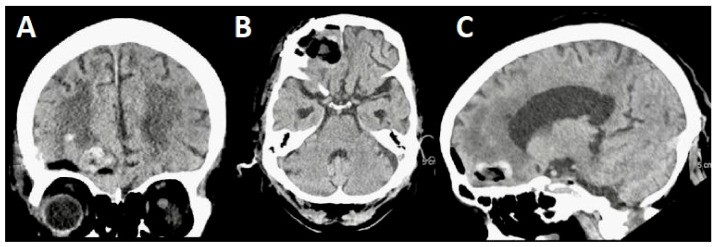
Case 2’s postoperative day 1 MRI showing a small amount of blood in the resection cavity with minimal compression of the frontal lobe. (A) Coronal view. (B) Axial view. (C) Sagittal view.
